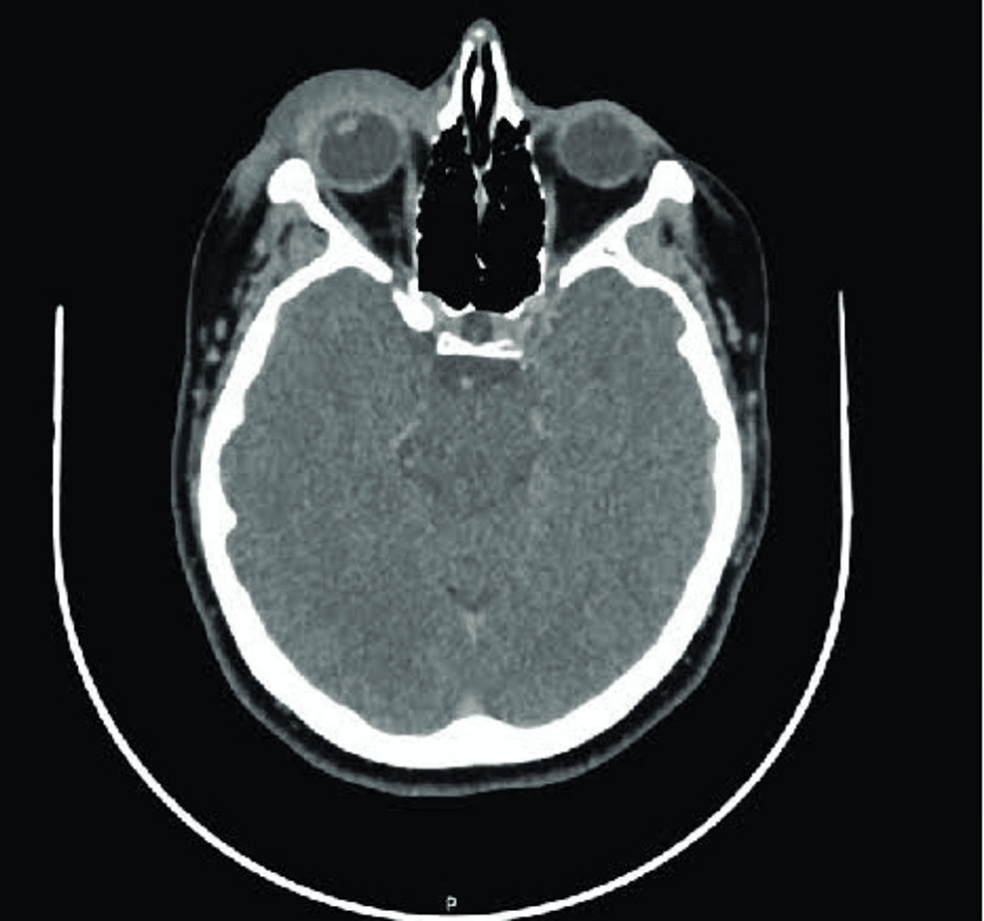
CT scan of the brain The image showed proptosis with diffuse soft tissue thickening anterior to the right orbital septum with thickening of the walls of the right eye suggestive of the thickening of ocular coats CT: computed tomography
